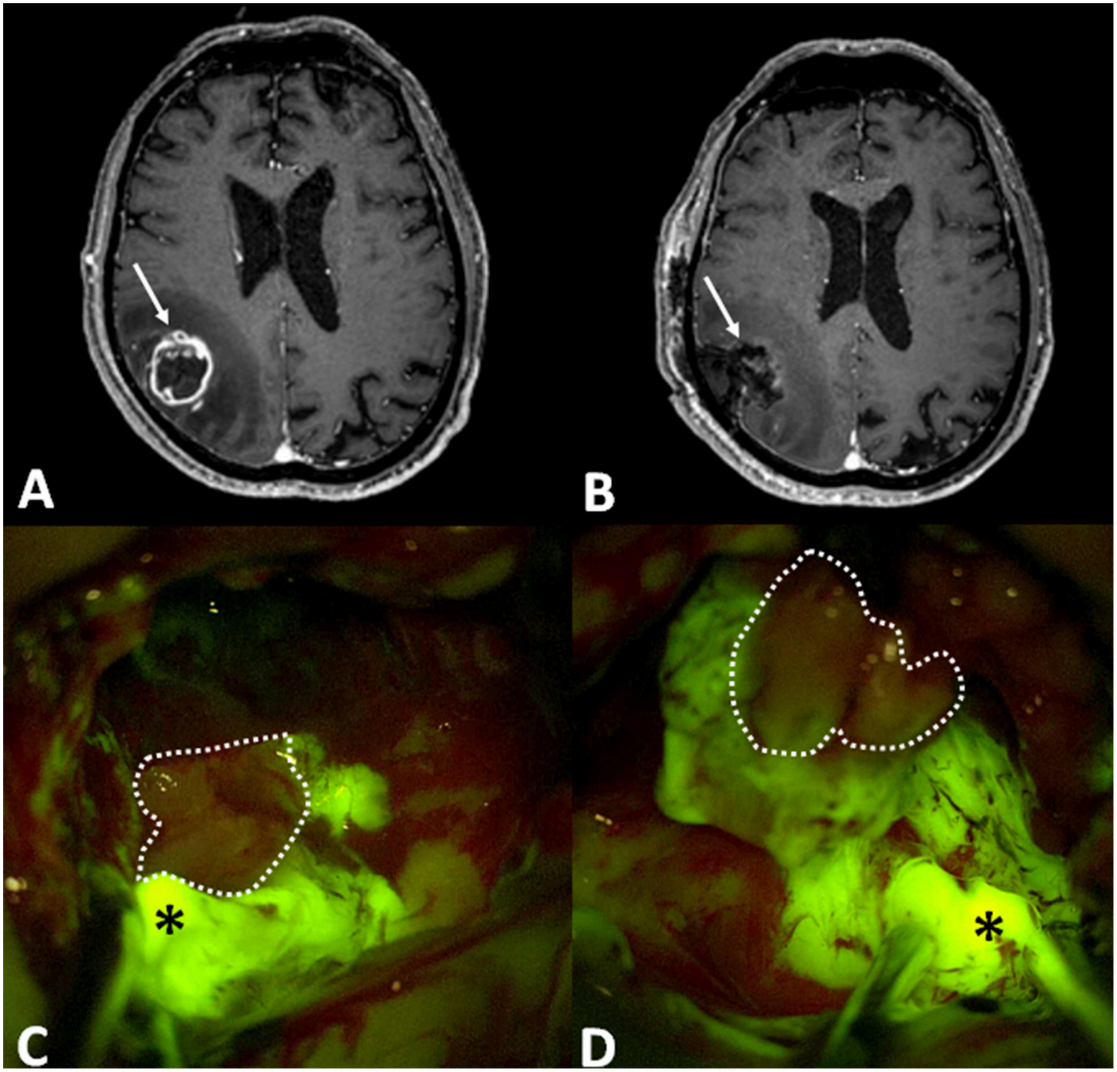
(A) Preoperative post-contrast axial T1-weighted MRI scan showing a right parietal GBM (arrow), that was completely removed, as visible in post-operative T1 with contrast axial scan (B: in that case arrow is the surgical cavity). Intraoperative images, under Y560 filter (C,D), depict bright yellow green signal (asterisk), in correspondence of nodular component, and dark pinkish feature in the necrotic core (dotted line).
